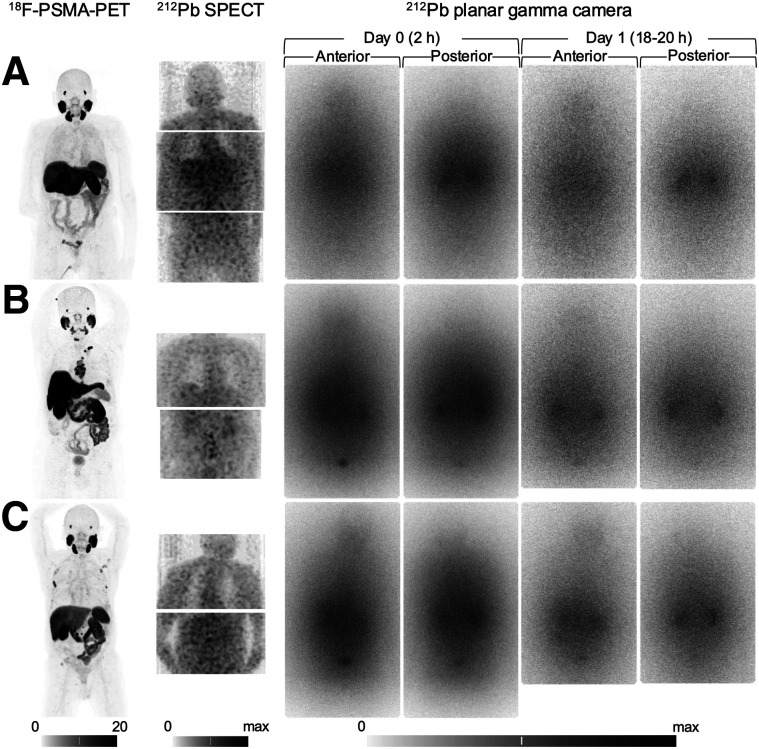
SPECT and planar γ-camera imaging of AB001: biodistribution compared with baseline ^18^F-PSMA PET for patients ID01 (A), ID02 (B), and ID03 (C). SPECT images show maximum-intensity projections with scaling of 0–maximum for each patient. PET images show maximum-intensity projections with scaling of 0–20 SUV_max_. WB γ-camera images are summed with scaling of 0–maximum.
